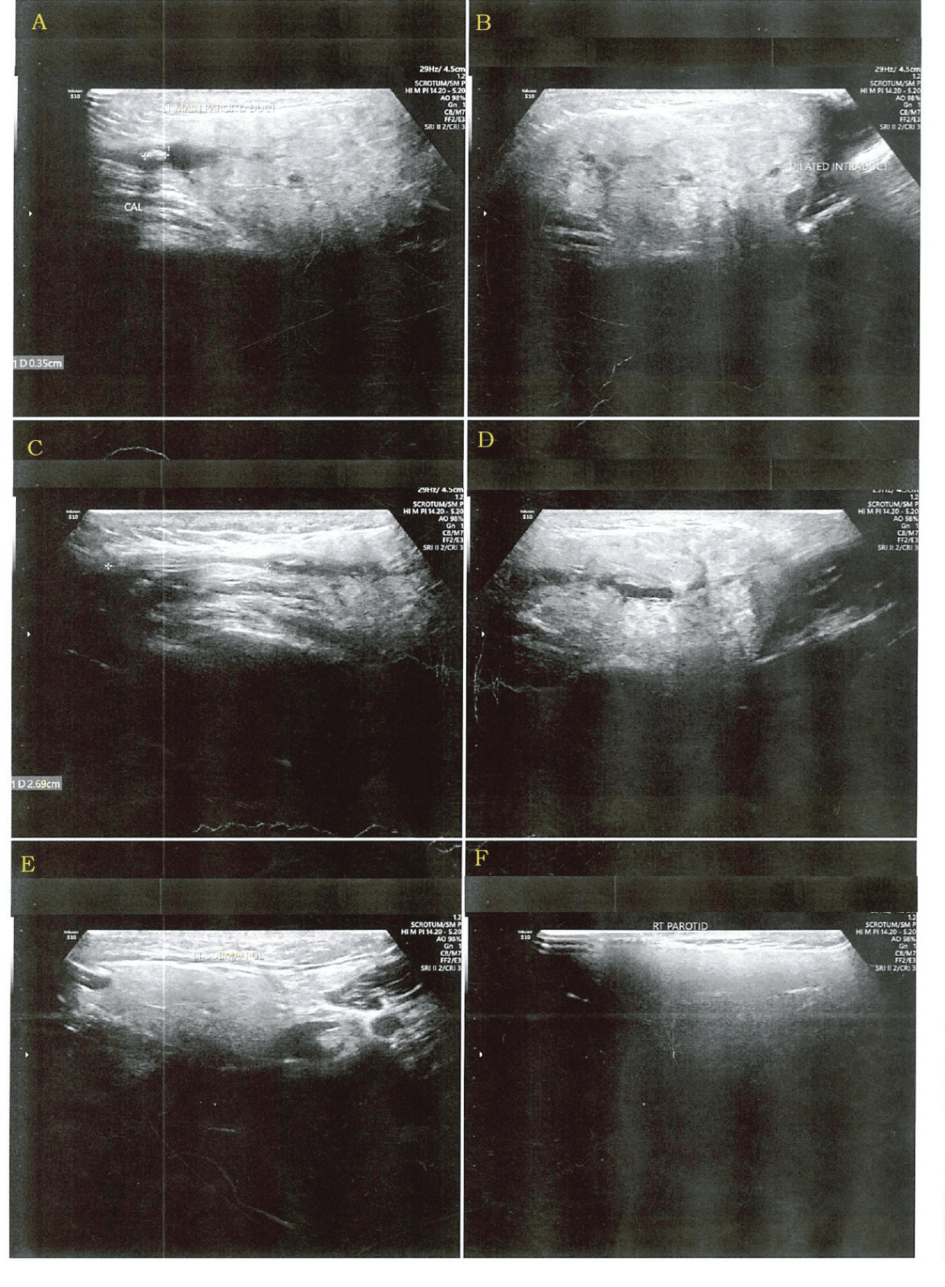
USG showing calculi USG: ultrasonography There are six boxes in the image. Each box is labeled as A, B, C, D, E, and F. A shows the calculi. The USG shows the main parotid duct calculi
